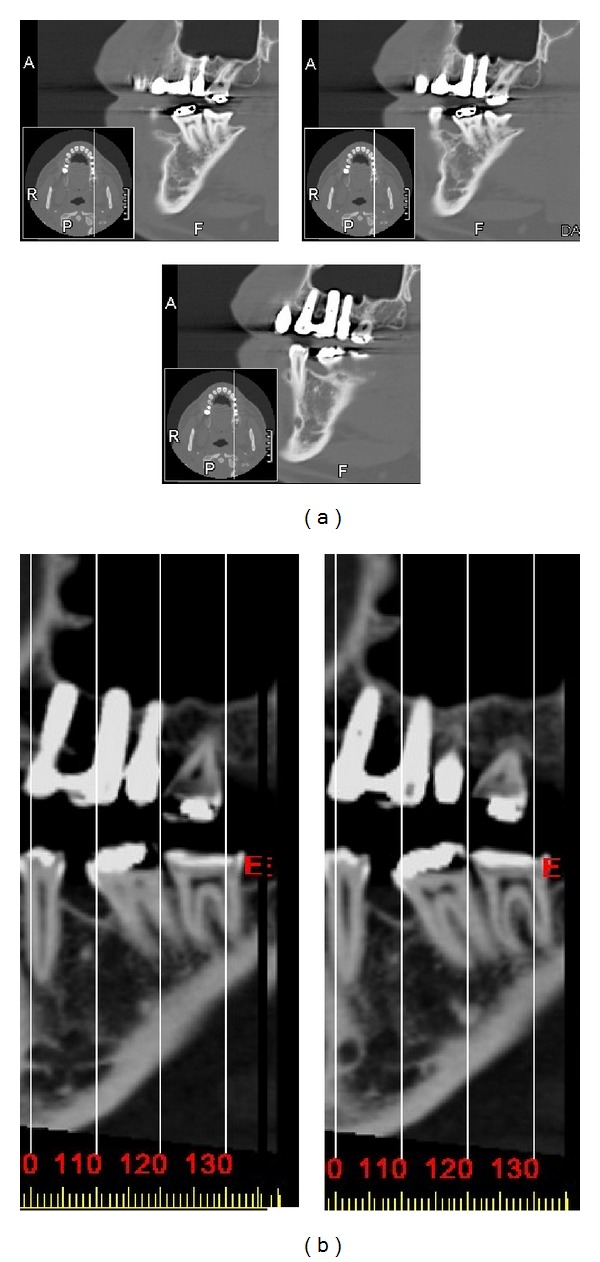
(a) CT scans showed the implant and surrounding bone and the new sinus floor using the staged osteotome sinus floor elevation technique on the left side 2 years after the operation. (b) Selected magnifying images for CT scans showed the implant, apical borders for these implants, surrounding bone, and the new sinus floor using the staged osteotome sinus floor elevation technique on the left side 2 years after the operation.
